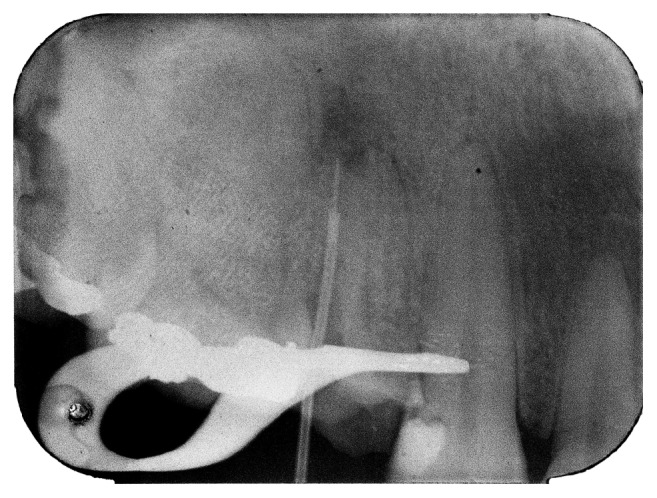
Periapical radiograph confirming the attachment of the fragment to the hypodermic needle.
